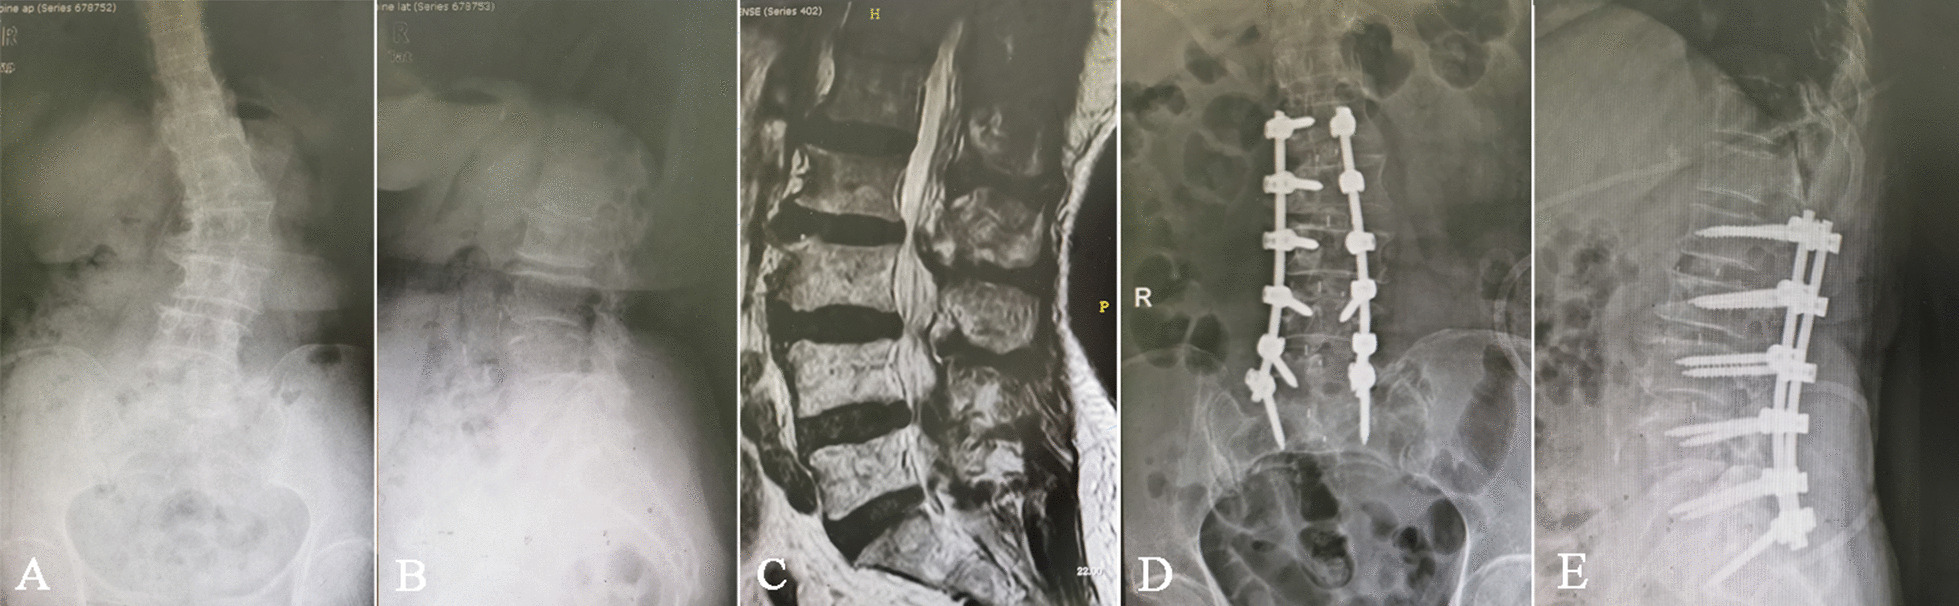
Preoperative lateral radiographic and MRI view (A–E) of a 72-year-old female with low back pain and numbness of the right lower limb for more than 3 years. Standing long cassette coronal and sagittal radiographs before surgery (A, B). The Cobb angle and lumbar lordosis were 26° and 42°, respectively. Standing long cassette coronal and sagittal radiographs at final follow-up after LSFCF surgery (D, E). The Cobb angle improved from 26° to 4°, and lumbar lordosis changed from 42° to 45°, respectively
